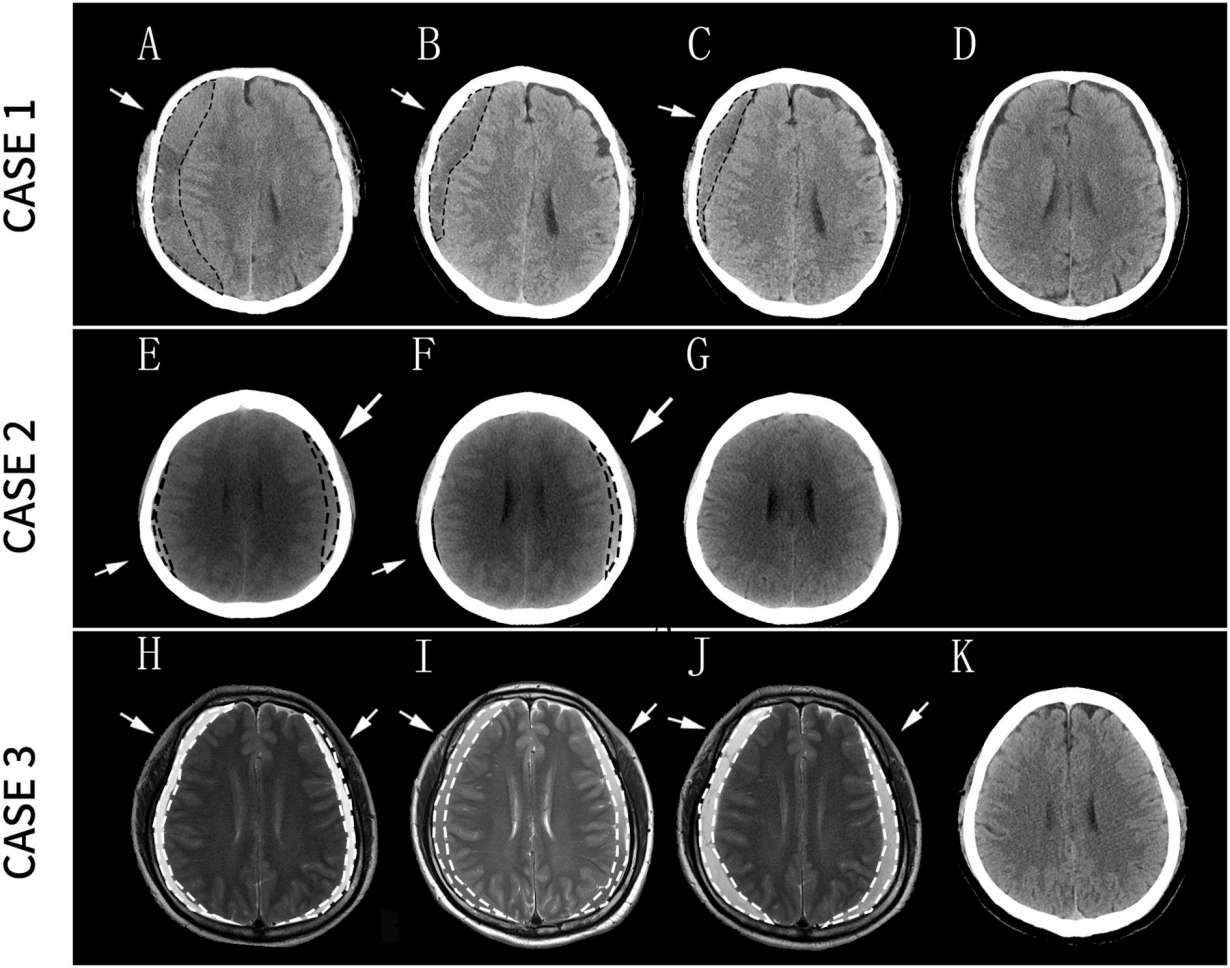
Case 1: (A) Diagnosis of right SDH; (B) The size of SDH significantly decreased after 9 days of the treatment; (C) SDH continued to decrease in size after 1 month of treatment; (D) the residual hematomas resolved 3 months after the treatment. Case 2: (E) Diagnosis of bilateral SDH; (F) the size of the right SDH significantly decreased after 1 month of the treatment; (G) the residual hematomas resolved 4 months after the treatment. Case 3: (H) Diagnosis of bilateral SDH; (I) Headache considerably decreased after 15 days of treatment but without a reduction in the size of the hematoma on MRI images; (J) Seventeen days later, a head MRI revealed an expansion of the SDH, and the patient underwent surgery after 2 days; (K) Two months later, the patient recovered well without any neurological sequelae. Arrows and dashed lines indicate the locations of SDH. SDH, subdural hematoma; MRI, magnetic resonance imaging.
